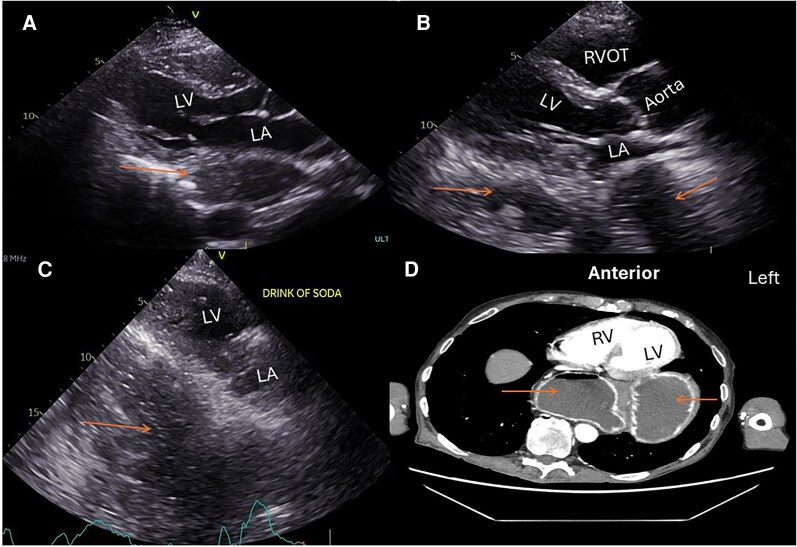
Parasternal long-axis view (*A* and *B*) showing a large structure (arrow) posterior to the left atrium causing extrinsic left atrium compression (*A*). Parasternal long-axis view (*C*) showing the structure posterior to the left atrium filled with soda bubbles (arrow) immediately after soda drink. Transverse computed tomography chest view at the cardiac level (*D*) showing the same structure with air level (arrows) posterior to the left atrium. The structure was part of the stomach in a patient with hiatal hernia. LV, left ventricle; LA, left atrium; RVOT, right ventricle outflow tract.
